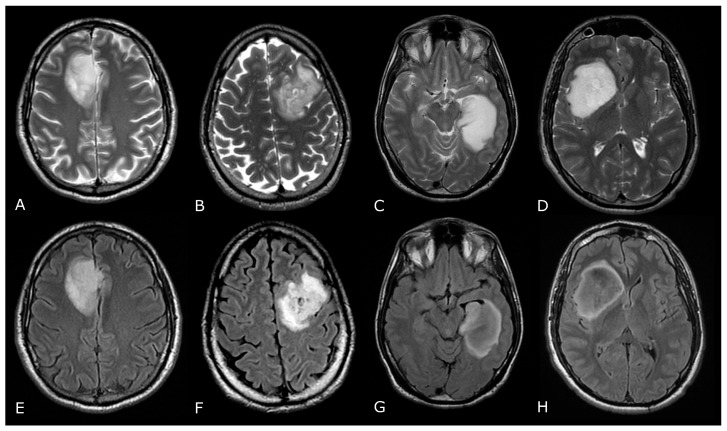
T2/FLAIR Mismatch Sign. Upper Row Ax T2w images, Lower Row Ax T2-FLAIR images. (A,E); (B,F) IDH-mut, 1p-19q codeleted ODs, respectively grade 2 (A,E) and grade 3 (B,F). (C,G); (D,H) IDH-mut, 1p-19q non codeleted Diffuse Astrocitomas, respectively grade 2 (C,G) and grade 3 (D,H). The T2-FLAIR mismatch sign (C) vs. (G) and (D) vs. (H) represents the T2 signal homogeneity of the mass with relatively hypointense signal throughout most of the lesion on FLAIR except for a peripheral rim of hyperintense signal. Notably, imaging features of grade 3 IDH-mut diffuse astrocytoma and OD may be indistinguishable from grade 2 IDH-mut diffuse astrocytoma and OD. However, grade 3 astrocytoma and OD may have more T2 signal heterogeneity (B,D).
